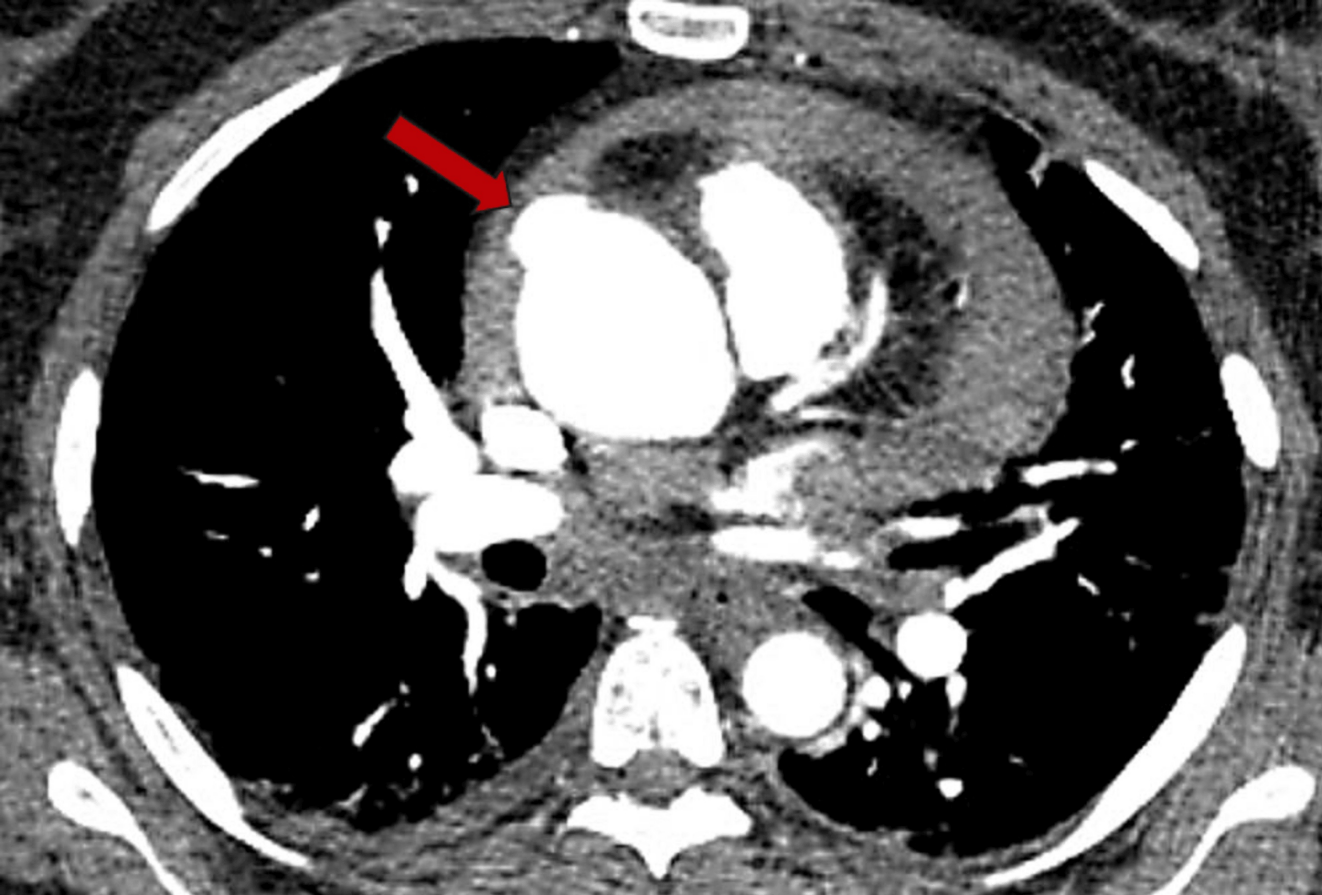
Axial CT chest angiogram revealing small ascending aortic aneurysm (red arrow).
